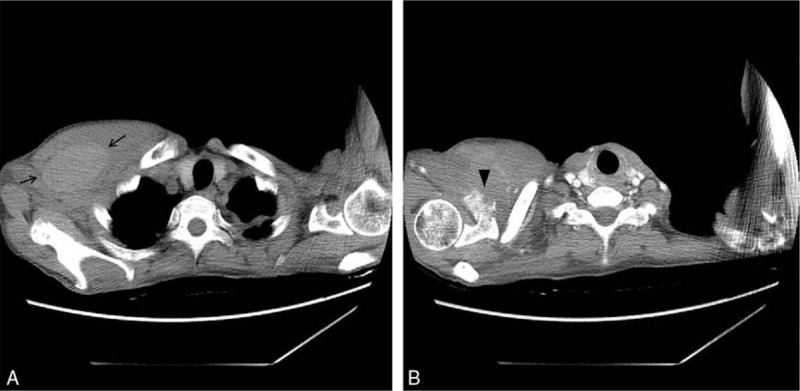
Chest computed tomography shows a highly attenuated large hematoma (arrow) within the right pectoral muscle of the right upper chest (A) and an ill-defined subtle enhancing exophytic metastatic mass in the right scapula with bony destruction (arrowhead), which caused the intramuscular hematoma (B).
